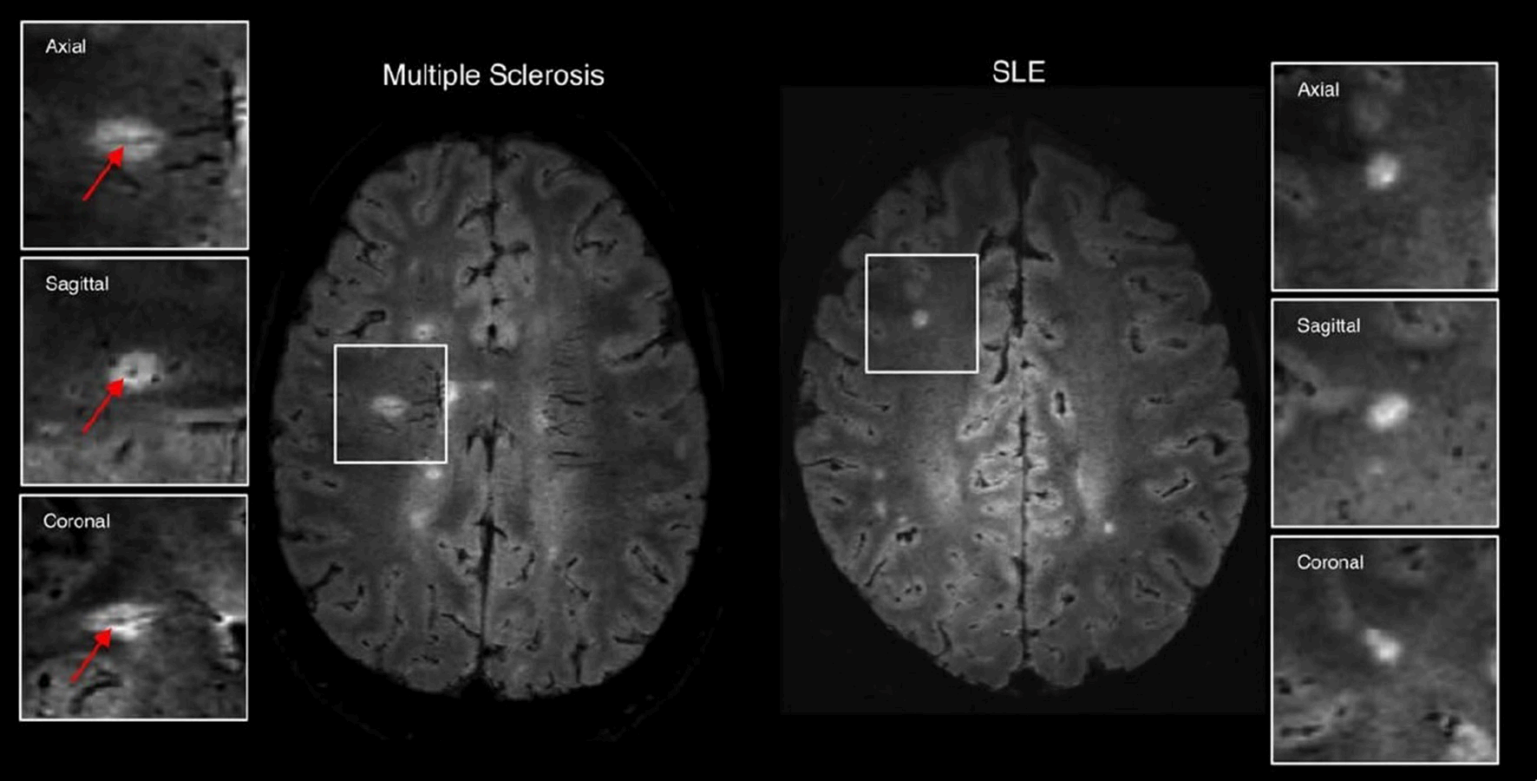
Examples of 3T FLAIR* imaging demonstrating the central vessel sign (arrows) in multiple sclerosis (left) but not in systemic lupus erythematosus (right). Figure adapted with permission from Maggi et al. (119).
